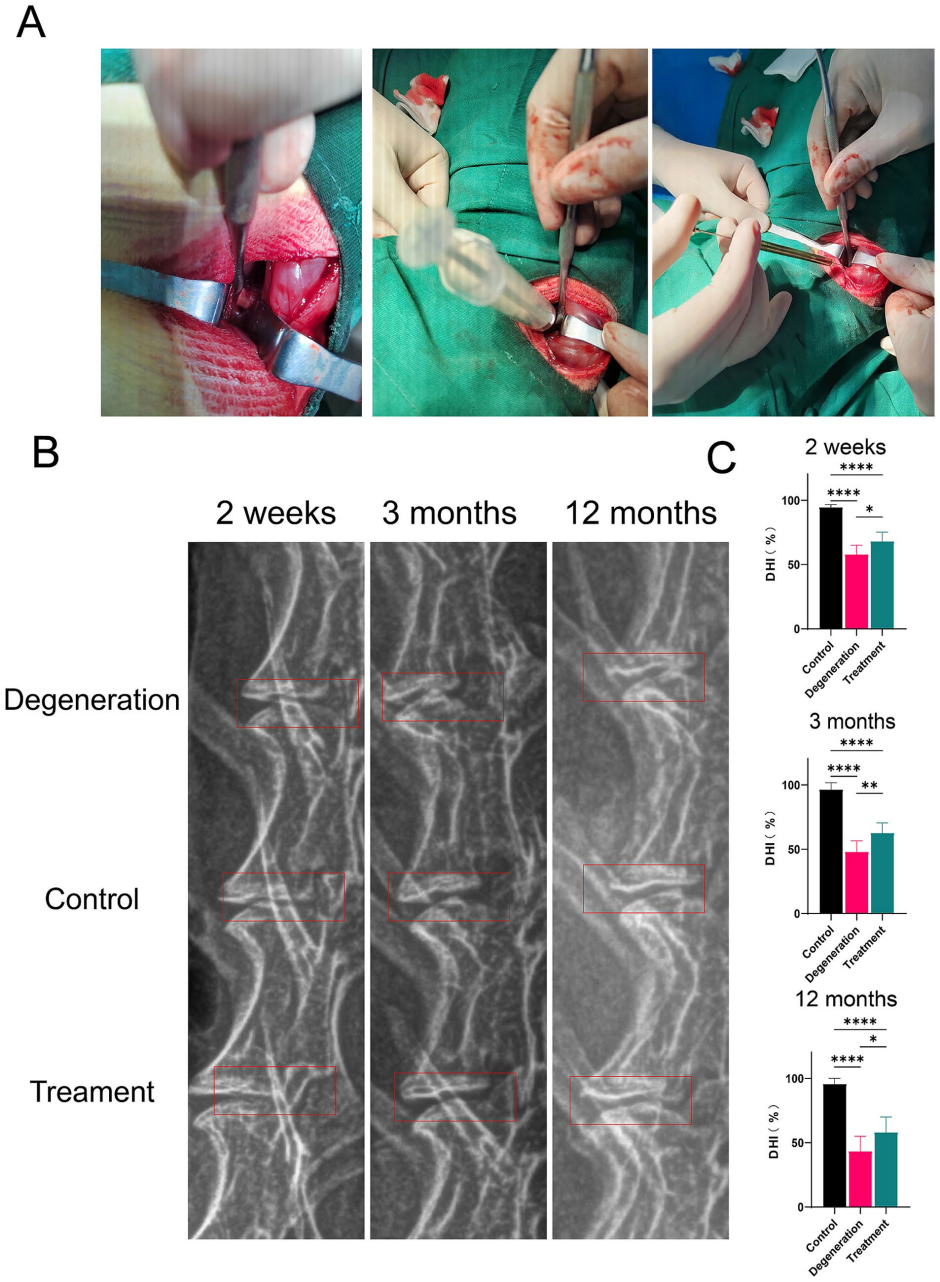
(A) Surgery photos. (B) Radiographic images. (C) The percentage of the disc height index (DHI). Error bars represent the S.D., and significant differences were assessed with Student’s *t* test; p* < 0.05, *p <* 0.01, p* < 0.001, and *p* < 0.0001.
